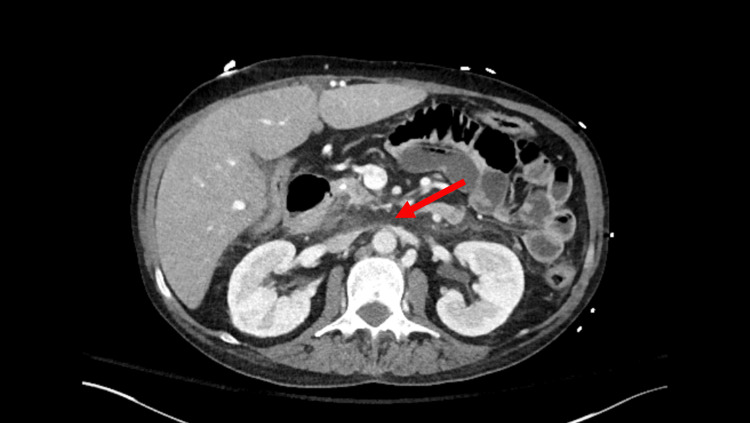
Computed tomography scan showing retroperitoneal fibrosis (red arrow).
